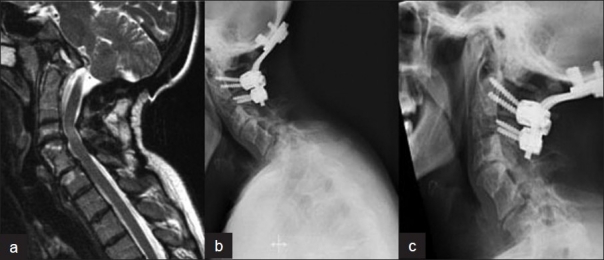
Mid sagittal T2WI (a) of cervical spine shows autofusion C5-6 with disc degeneration at C4-5 and C6-7. Lateral X-rays of cervical spine, flexion (b) extension (c) views show occipito cervical fusion performed before and some residual motion in the nonfused segment
